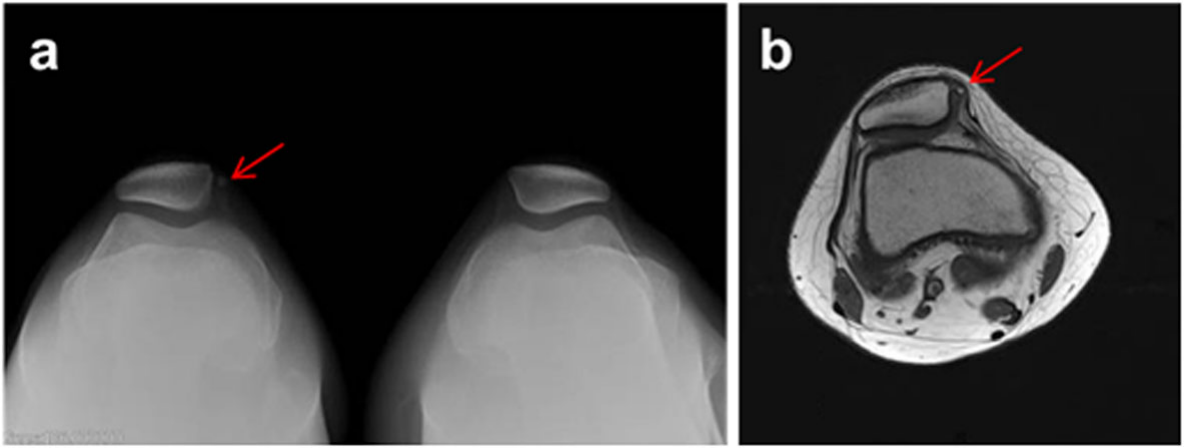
a A 15-year-old male sustained after an APD with bony avulsion on the medial patellar margin. A, Merchant view showing a bony avulsion (arrow) beside right patella medial margin. b Axial T2-weighted image showing a fracture fragment on medial aspect of the patella (arrow)
